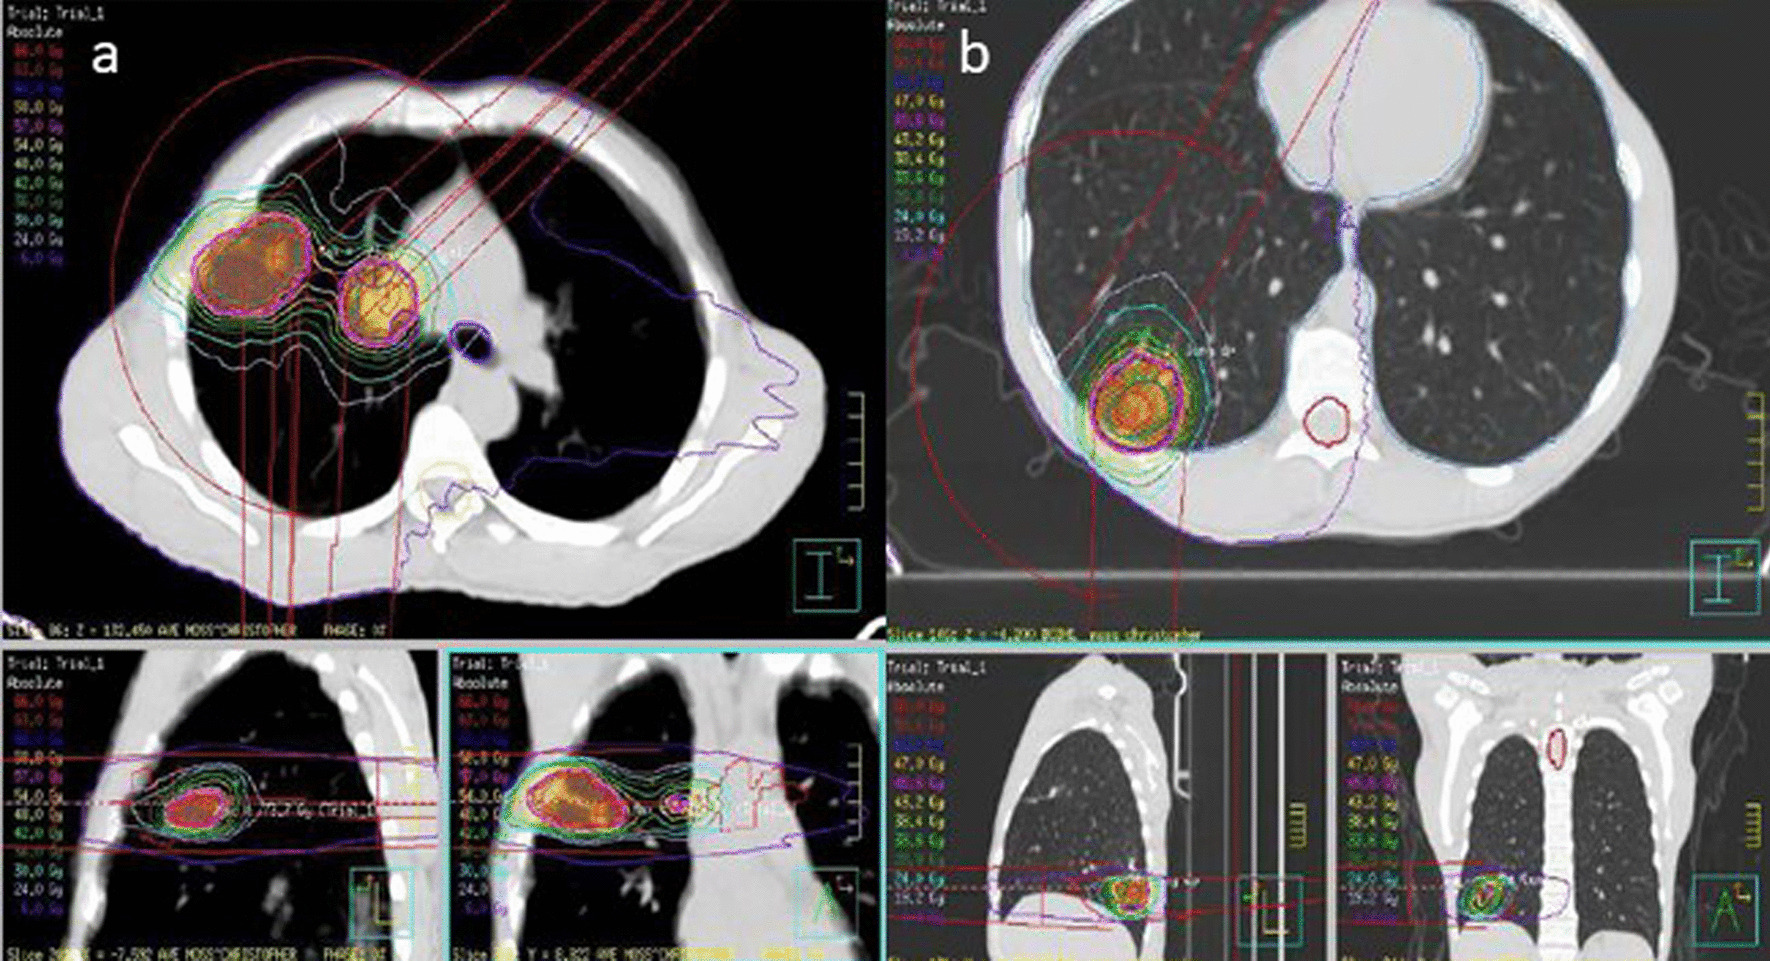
Computed tomography planning scan and isodose lines representing radiation dose distribution. a Planning target volumes 1 and planning target volumes 2 (hilar nodal bed and right upper lobe tumour bed, respectively). b Planning target volumes 3 (right lower lobe tumour bed)
